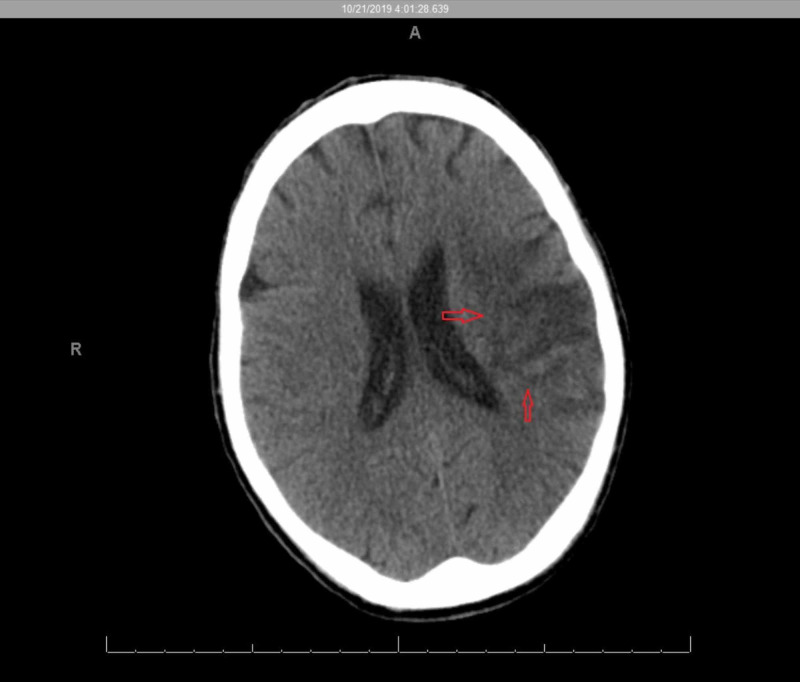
CT of the head without contrast showing middle cerebral artery infarct (red arrows)
